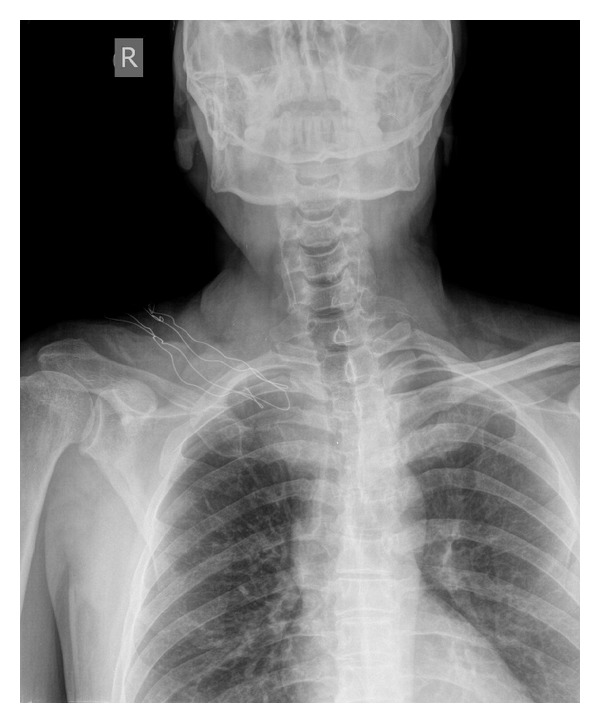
Anteroposterior roentgenography after removal of right clavicle.
